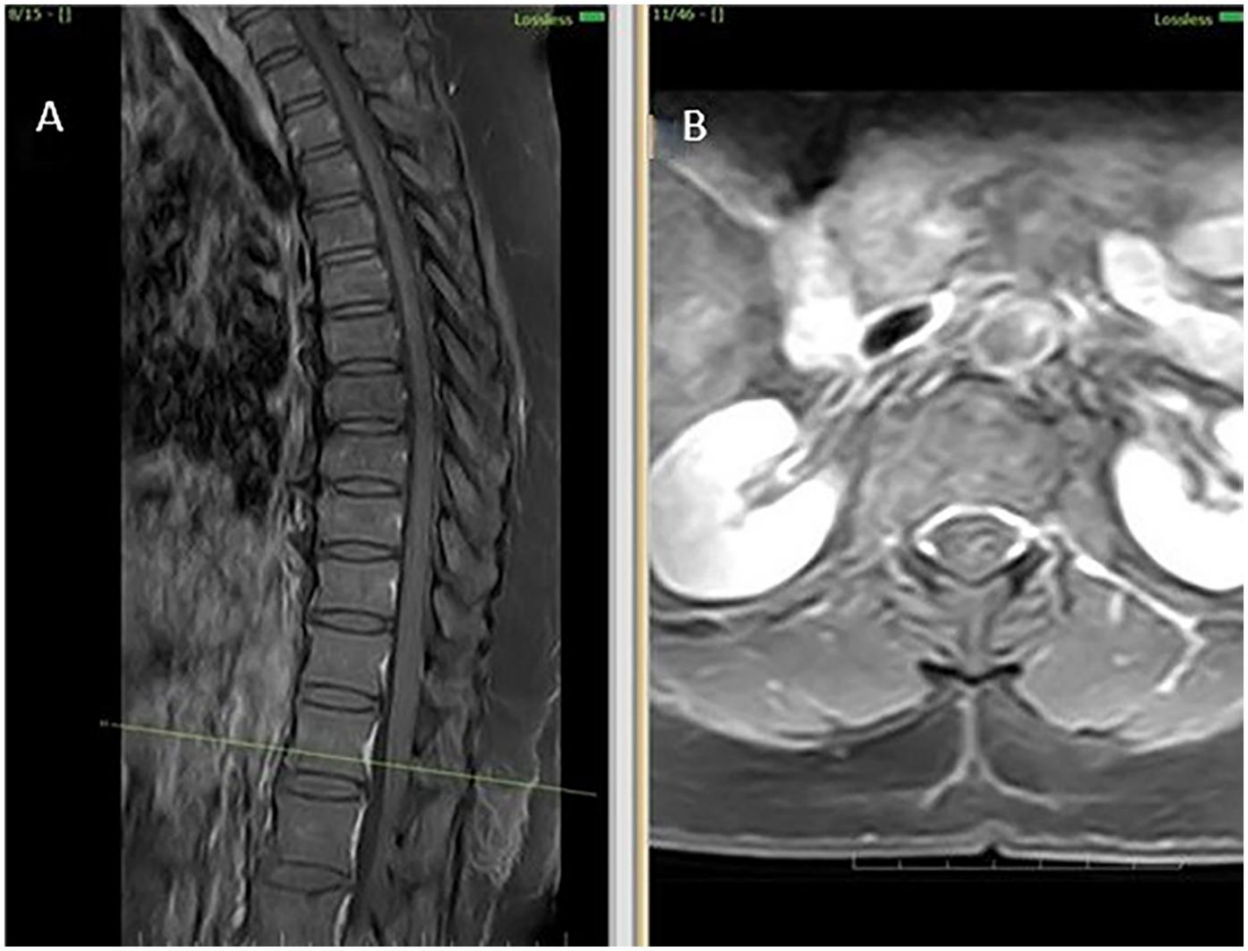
MRI thoracolumbar spine findings suggestive of sulcal artery infarction. (A) Sagittal post-contrast T1-weighted image reveals no contrast enhancement at the lesion site, ruling out inflammatory or neoplastic causes. (B) Axial post-contrast T1-weighted MRI confirms absence of mass effect or enhancement, supporting a non-compressive vascular etiology.
